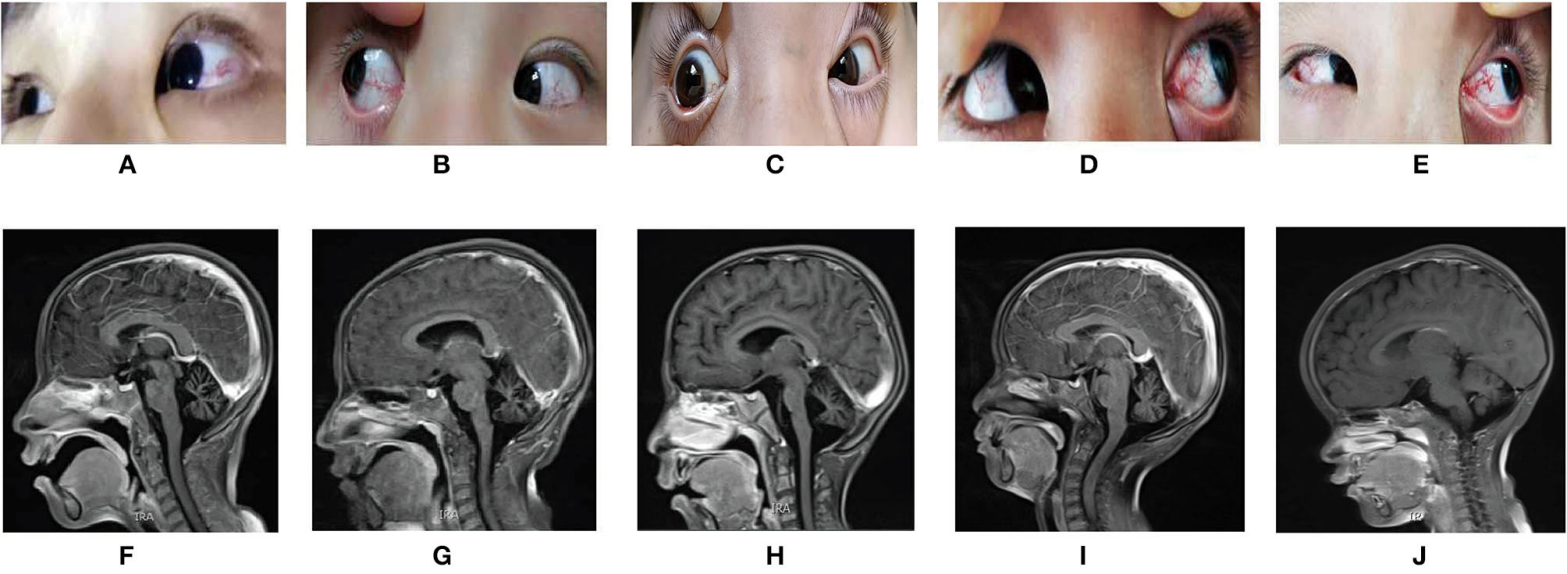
(A–E) showed the bulbar conjunctival telangiectasia of the five AT patients; (F–J) were the pictures of the Brain MRI of the five AT patients, all showed the cerebellar atrophy. (A) and (F) are from case 1, (B) and (G) are from case 2, (C) and (H) are from case 3, (D) and (I) are from case 4, (E) and (J) are from case 5.
